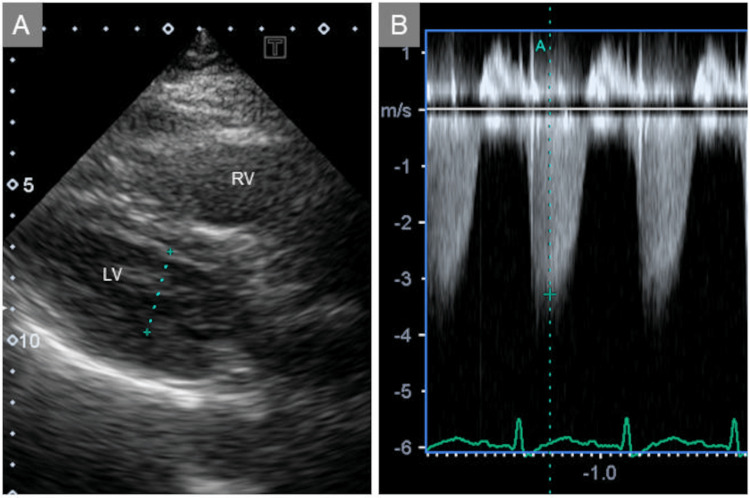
Transthoracic echocardiographic images Transthoracic echocardiography revealed right ventricular enlargement (A) and moderate tricuspid regurgitation with a peak velocity of 3.2 m/s (B). RV, right ventricle; LV, left ventricle
